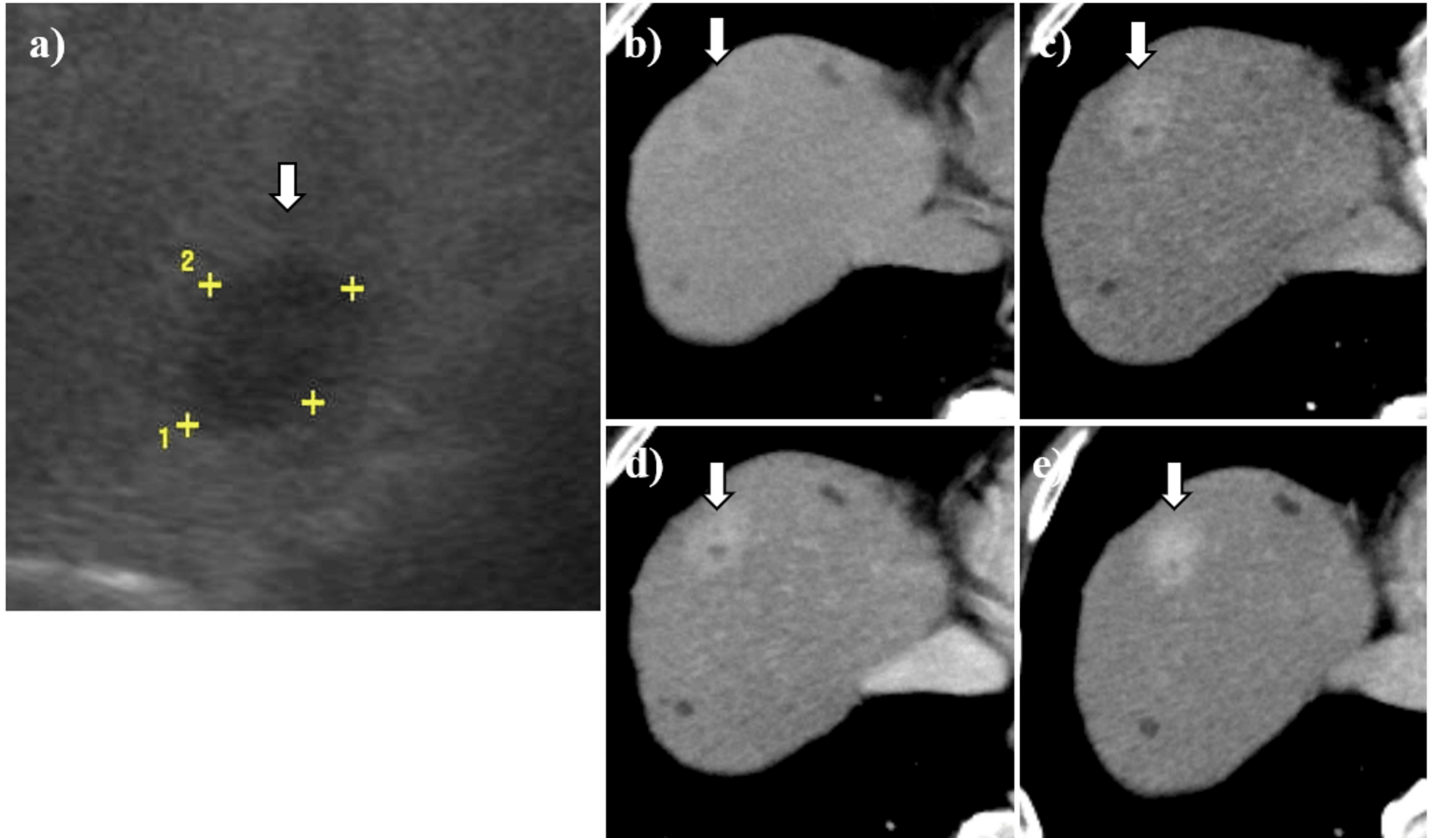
The imaging of abdominal ultrasound sonography and computed tomography. (a) Abdominal ultrasound sonography showed the hypoechoic area (arrows) (21 mm × 15 mm in diameter) as a background with hyper-echogenicity due to fatty liver change. (b) Abdominal plain CT showed a lower density nodule with slightly higher density hazy border (arrows). (c) Contrast-enhanced abdominal CT in the arterial phase showed hyperenhancement effect for the hepatic nodule with a part of inner non-enhancement area (arrows). (d) and (e) The hyperenhancement effect of the hepatic nodule continued in portal and equilibrium phase (arrows).
